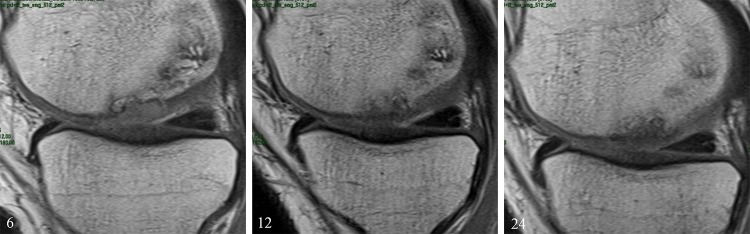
Magnetic resonance imaging evaluation of a coll-HA implant in the medial femoral condyle, showing ongoing osteochondral remodelling at 6 months, 1 and 2 years of follow-up
